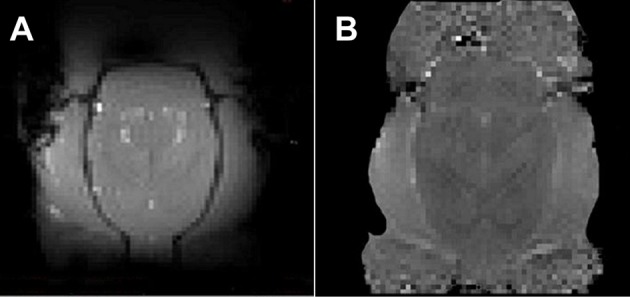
pH-weighted APT magnetic resonance imaging of a normal subject at 7.0 T. (A) MT image obtained with RF applied at −3.5 and 3.5 ppm (1,050 Hz at 7.0 T), and without RF irradiation. (B) The APT imaging was obtained with RF irradiation and via processing with Vnmr J 3.1A and Matlab software, which provide high spatial resolution and a homogeneous signal, and enable resolution of the cerebrum anatomy. APT, amide proton transfer; RF, radio frequency.
